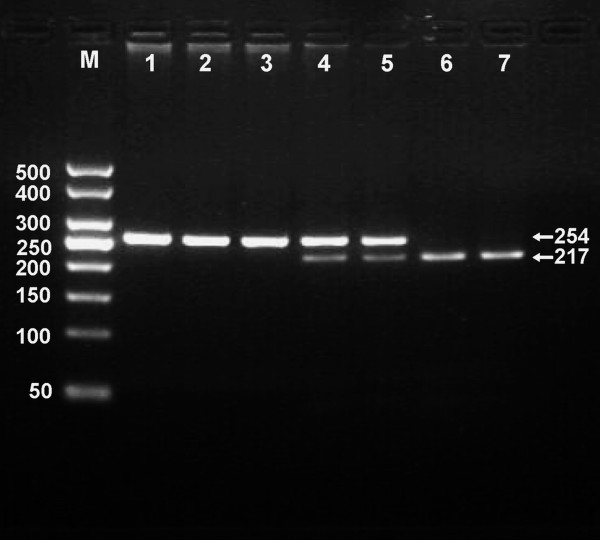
Genotyping of the *LIPG *584C > T polymorphism. Lane M, 50 bp Marker Ladder; lane 1, the PCR product of the sample (254 bp); lanes 2 and 3, CC genotype (254 bp); lanes 4 and 5, CT genotype (254-, 217- and 37-bp); and lanes 6 and 7, TT genotype (217- and 37-bp). The 37 bp fragment was invisible in the gel owing to its fast migration speed.
